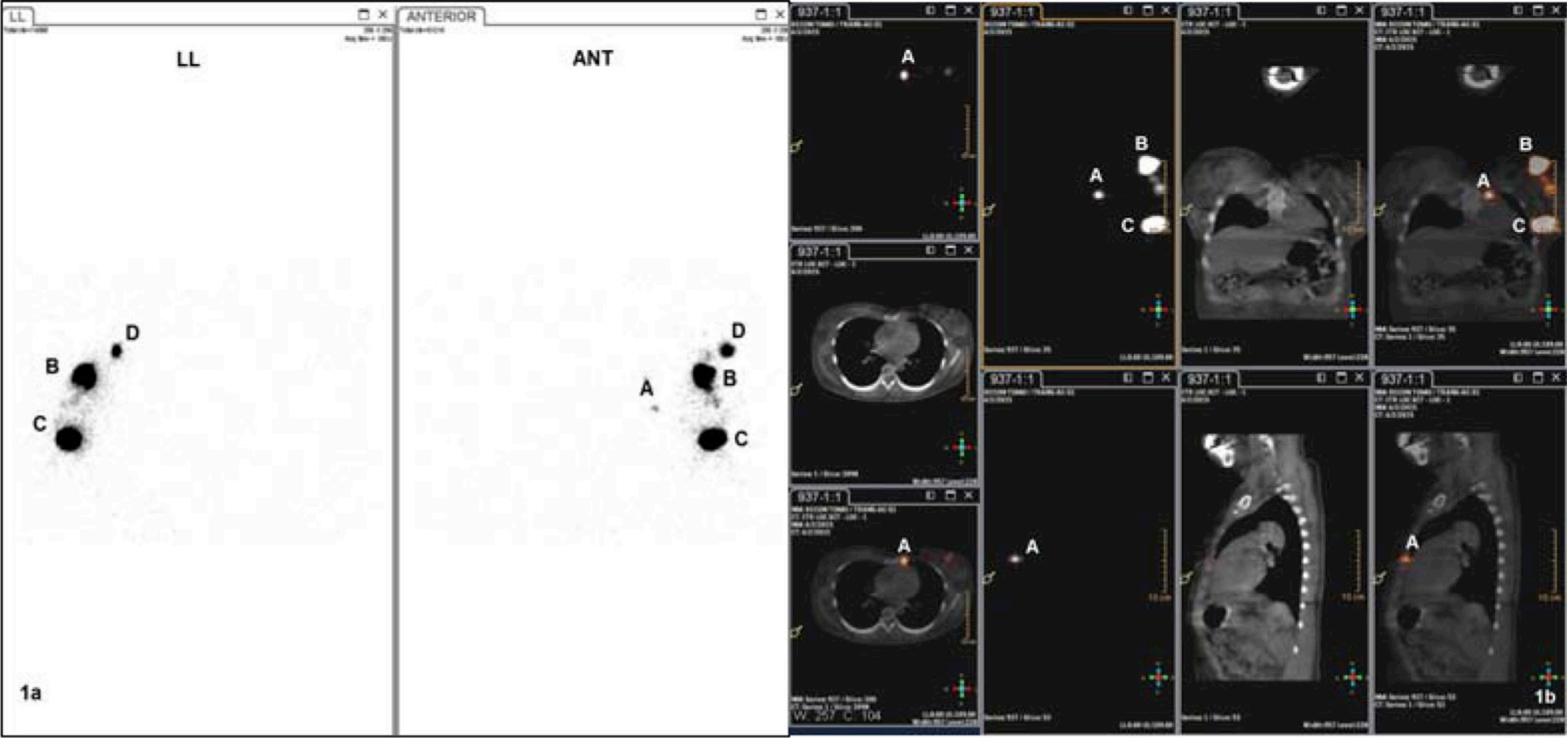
SPECT(1a) and SPECT/CT(1b) image before operation A: radioactive IM-SLN, B and C: injection point, D:radioactive ASLN, ANT: anterior imaging, LL: left lateral imaging
